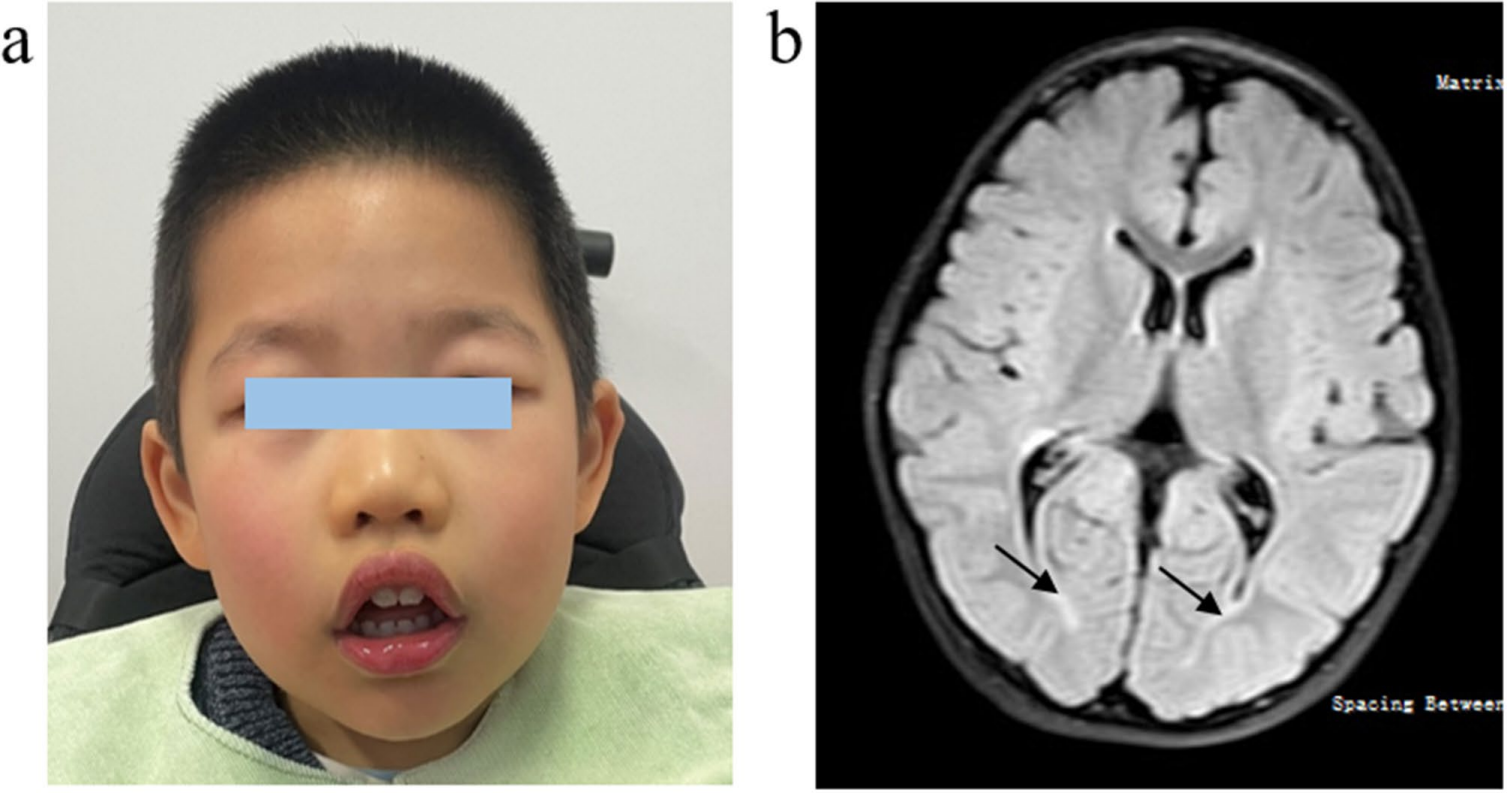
Clinical manifestations. a Face picture of the patient. b Brain MRI of the patient. T2 FLAIR showing a small abnormal signal in the bilateral posterior horn of the lateral ventricles (arrows)
